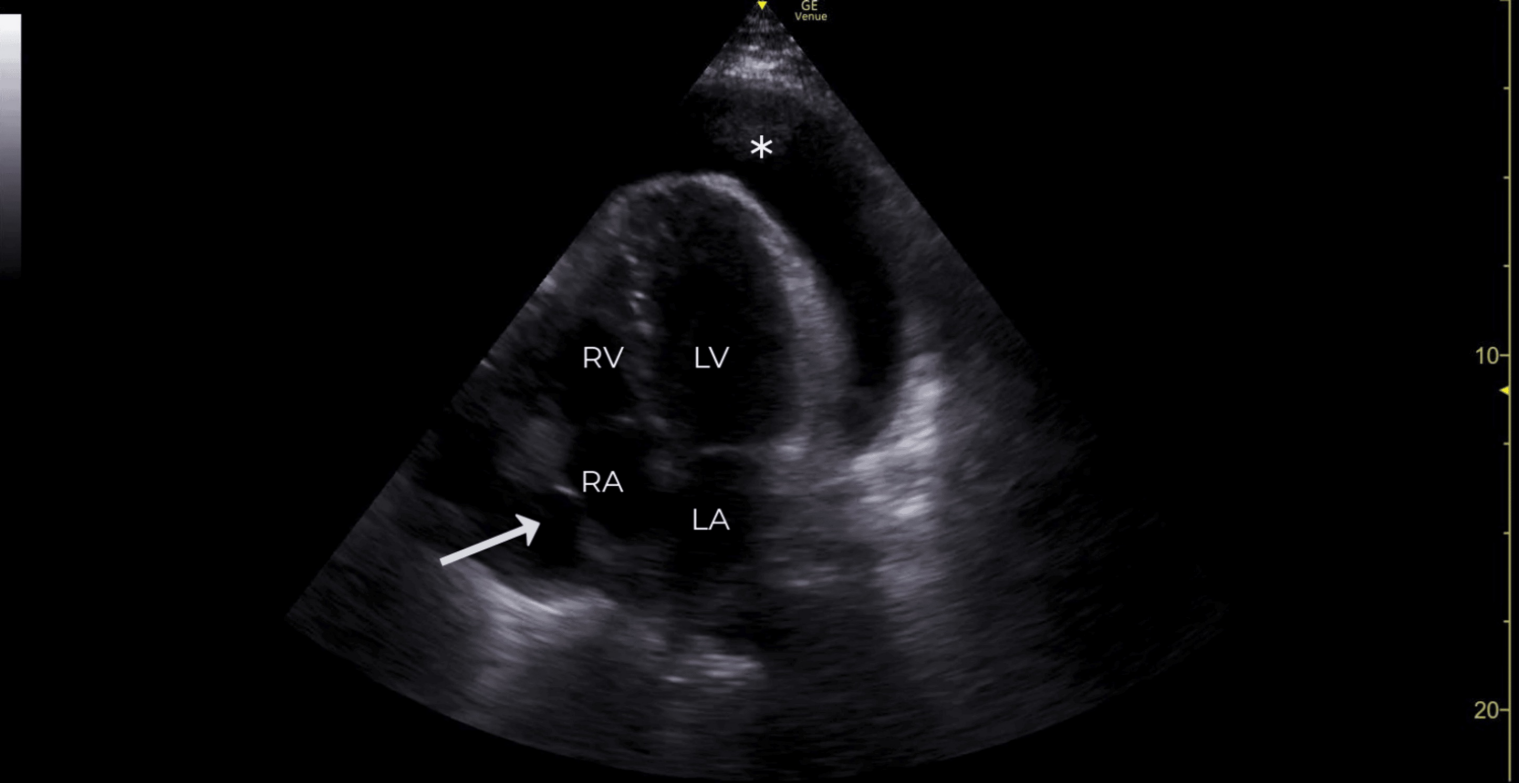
Apical four-chamber view with large pericardial effusion (*) causing systolic collapse (arrow) of the right atrial (RA) wall consistent with tamponade
